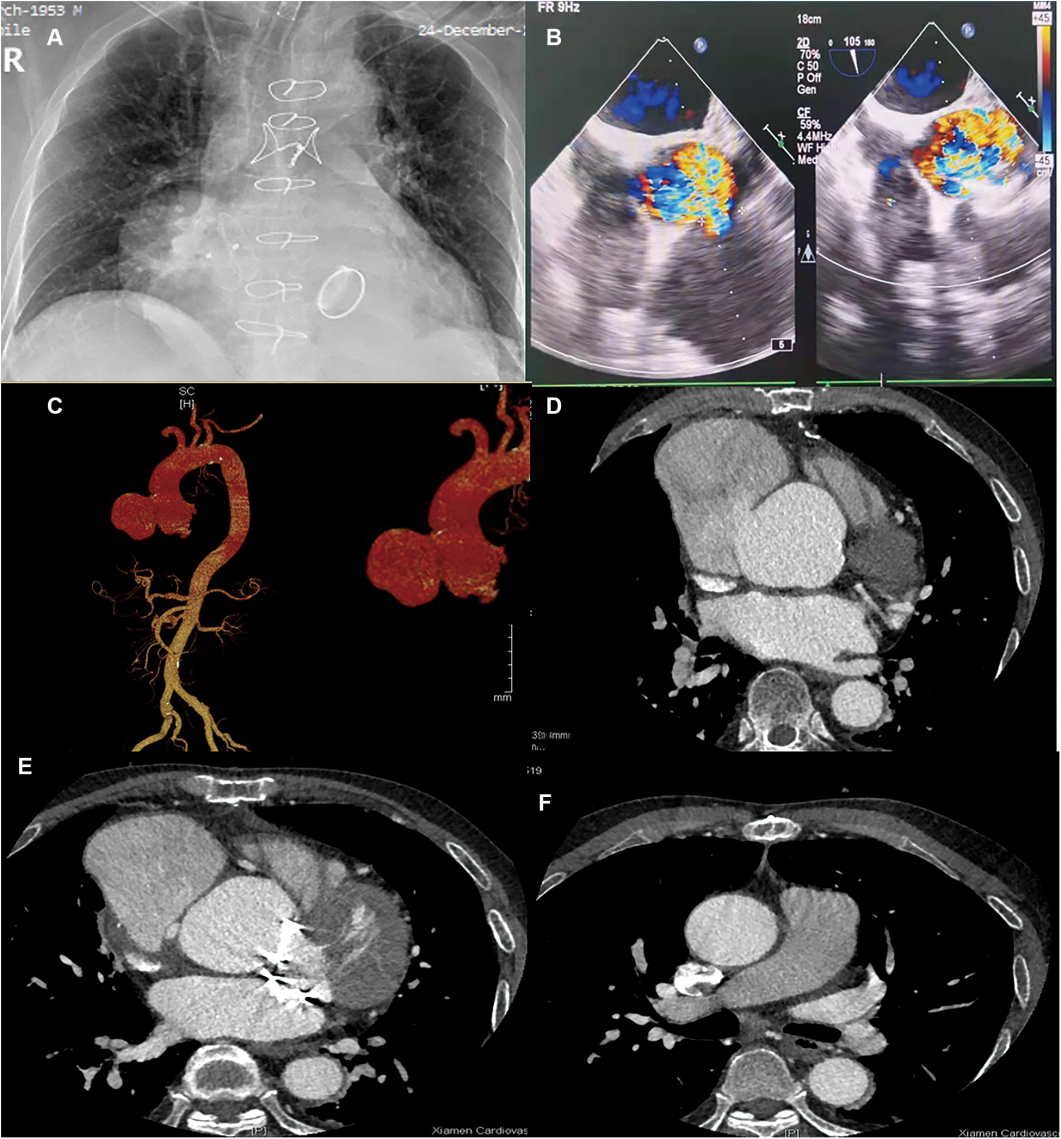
Preoperative: (A) Chest ray; (B) Cardiac ultrasound; (C–F) CT of coronary artery + thoracic and abdominal aorta [(C) Front position; (D) Tumor waist; (E) Lower border of the tumor; (F) Pulmonary bifurcation].
